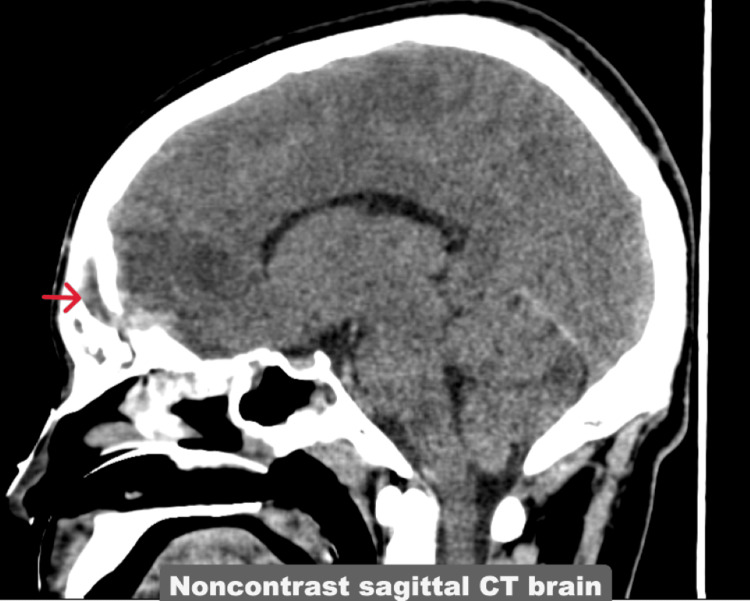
Day 2 non-contrast axial CT brain showing persistent right frontal sinus hemorrhage Red arrow shows persistent right frontal sinus hemorrhage.
